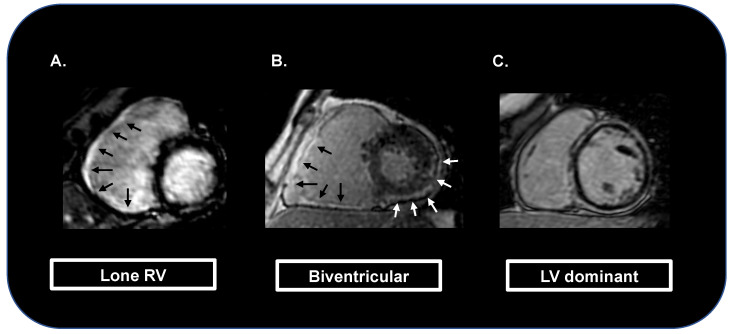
Cardiac magnetic resonance images showing myocardial fibrosis (as late gadolinium enhancement areas) limited to the right ventricle (black arrows; A), also extended to the left ventricle (white arrows; B), or with dominant left ventricular involvement («ring-like» late gadolinium enhancement pattern; C).
